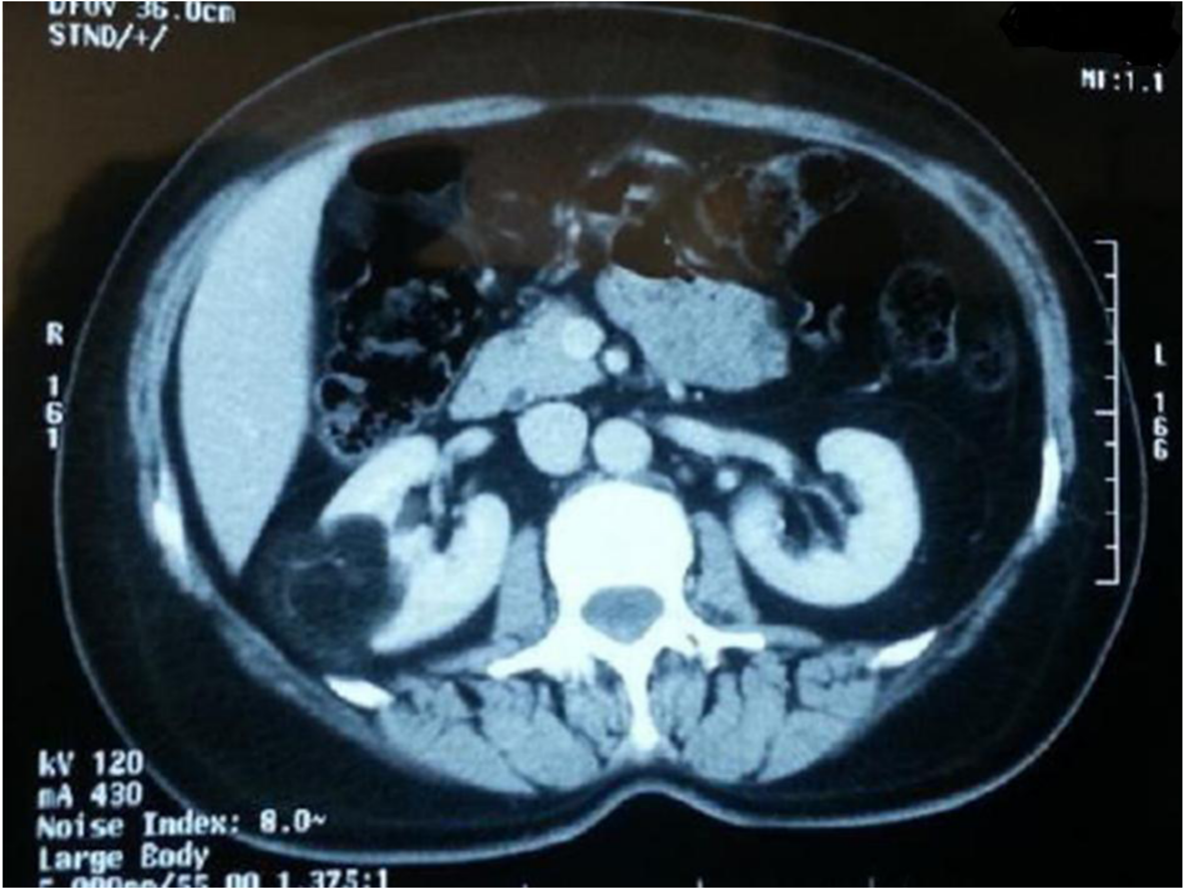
Subsequent computed tomography after six months revealing quasi-normal aspect of kidneys.
